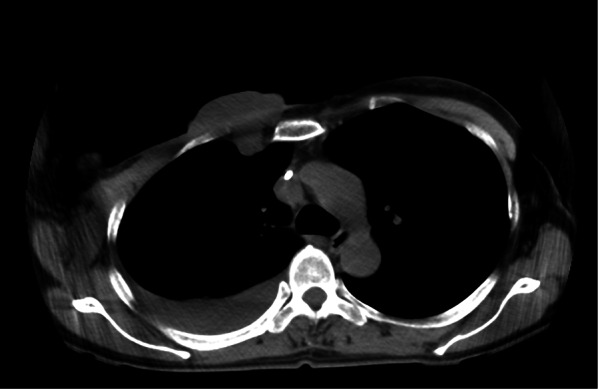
Computed tomography showed a mild reduction in chest wall tumor and pleural dissemination after one cycle of AI therapy
